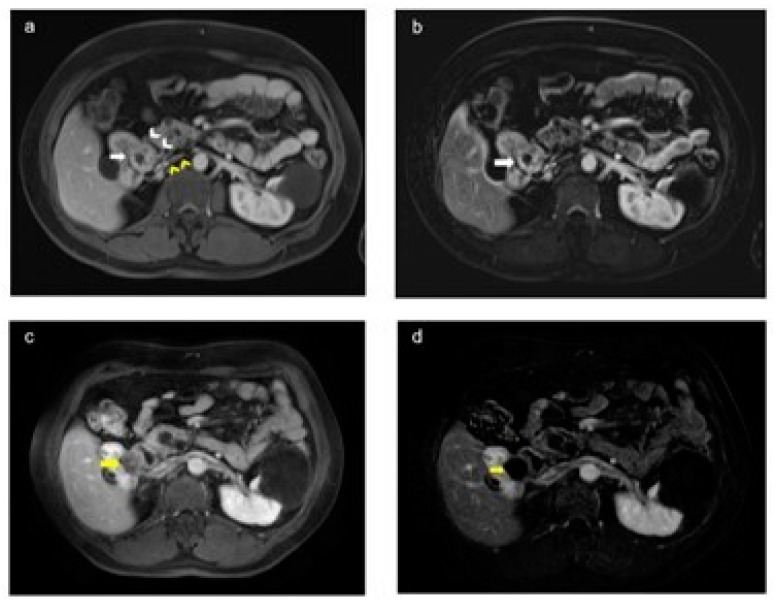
Preoperative and postoperative magnetic resonance (MR) images in a patient with von Hippel–Lindau (VHL) syndrome (a,b). The axial plan postcontrast portal venous phase and T1-weighted images show a preoperative lesion (white arrow) in the upper pole of the right kidney, in close proximity to renal vascular structures (yellow arrowhead) and the intestine (white arrowhead) (c,d). The axial postcontrast portal venous phase and subtracted T1-weighted images show no residual lesion in the tumor bed (yellow arrow) after laparoscopy-assisted ultrasound-guided perioperative microwave ablation therapy.
